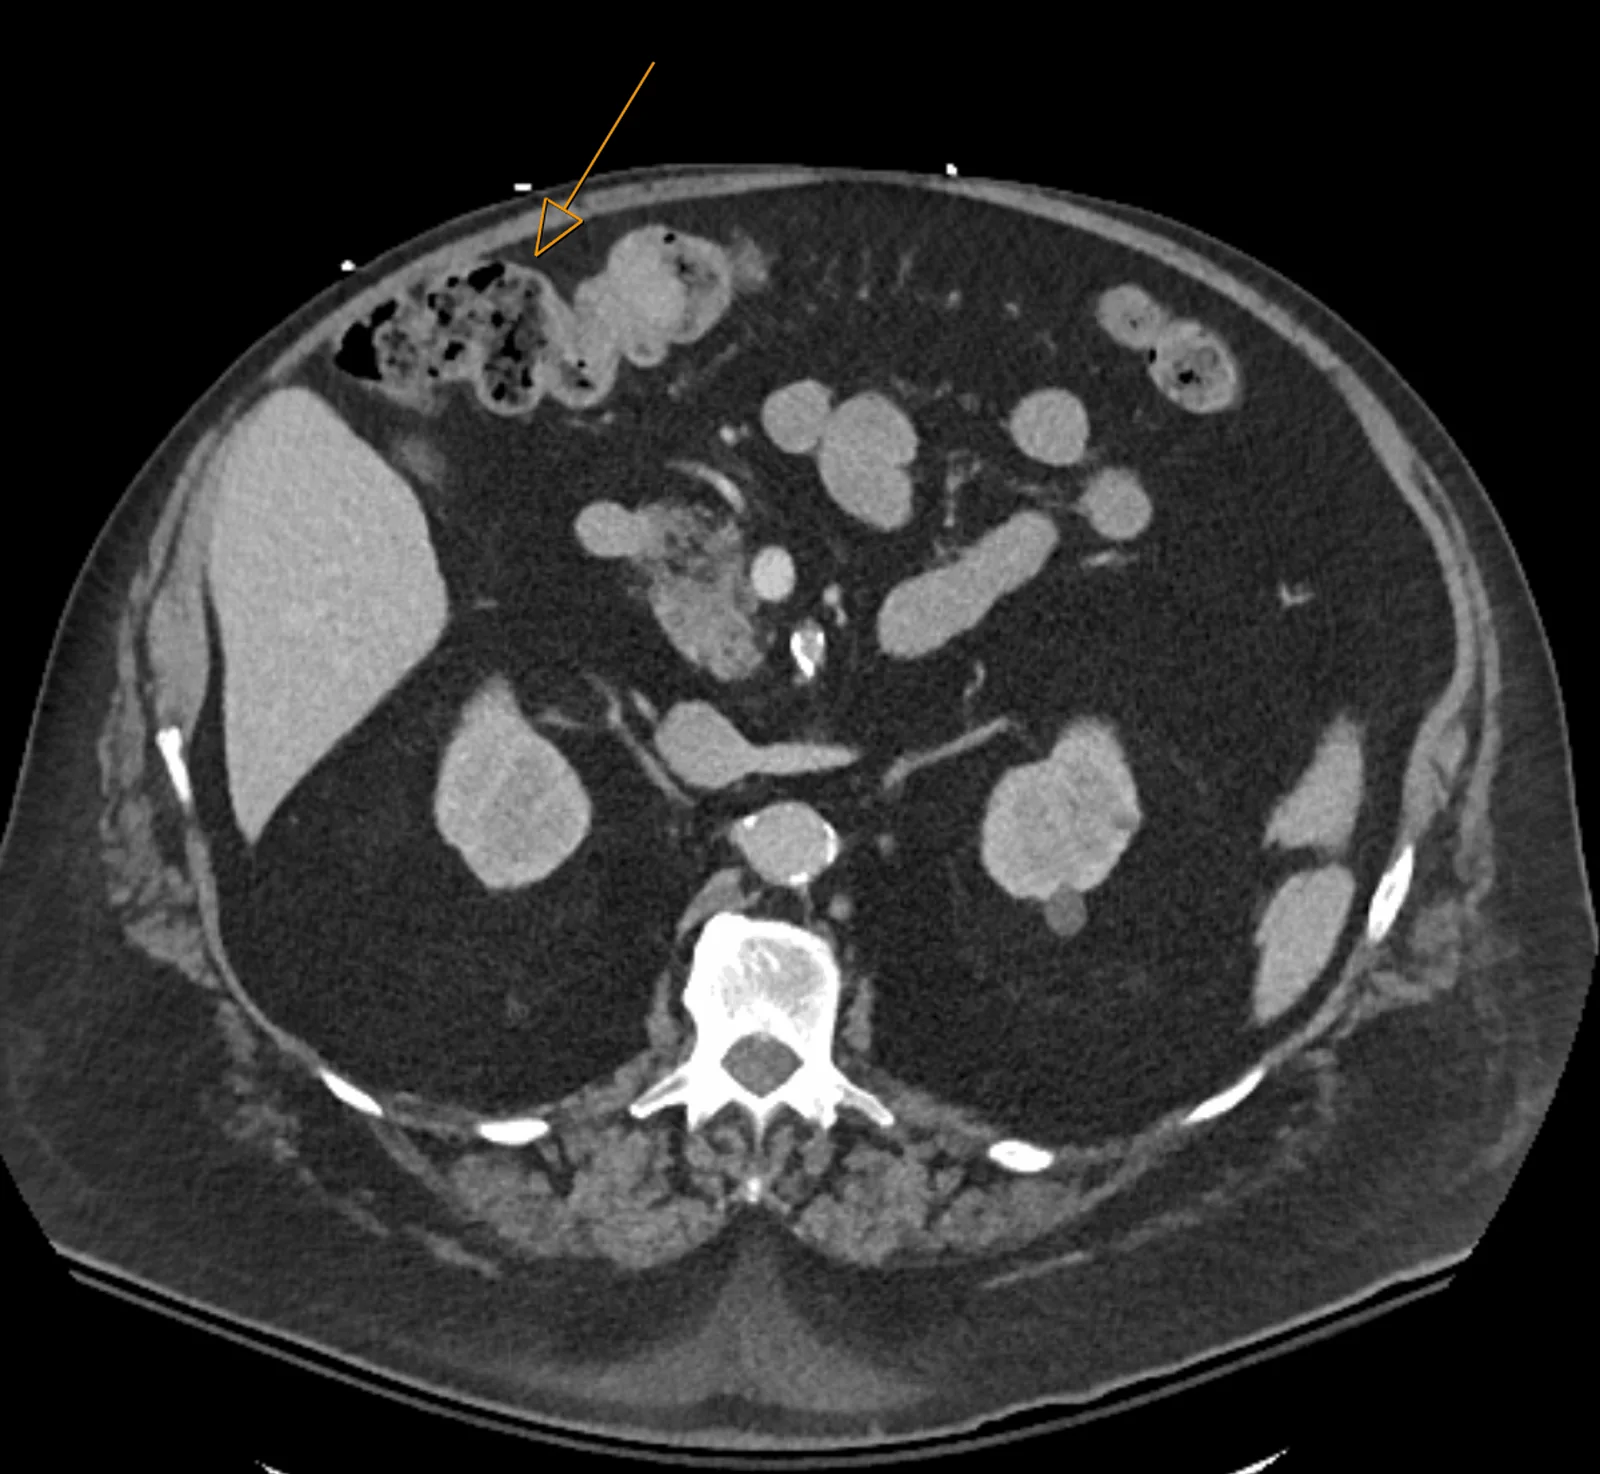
Computed tomography of the abdomen demonstrating a 2.9 cm by 2.9 cm transverse colon mass (arrow).
